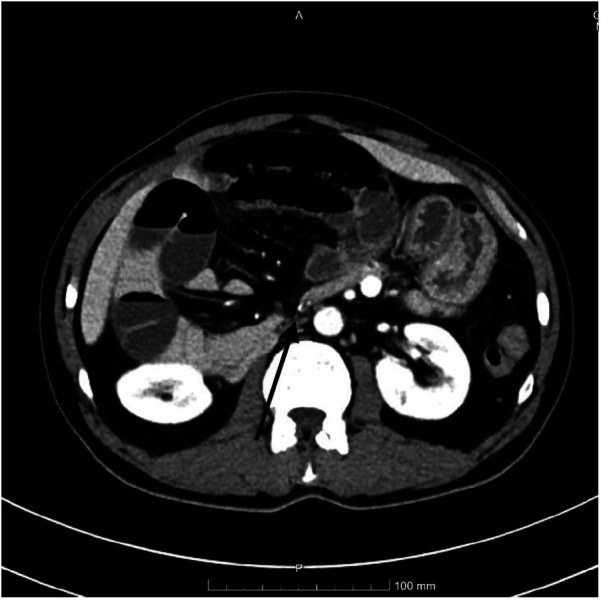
Contrast-enhanced abdomen/pelvis CT scan. A tightly narrowed transition point (2.7 cm) in the mid-abdomen, with proximally dilated small bowel loops and collapsed distal bowel, consistent with mechanical small bowel obstruction. The paraduodenal hernia defect is not directly visualized.
